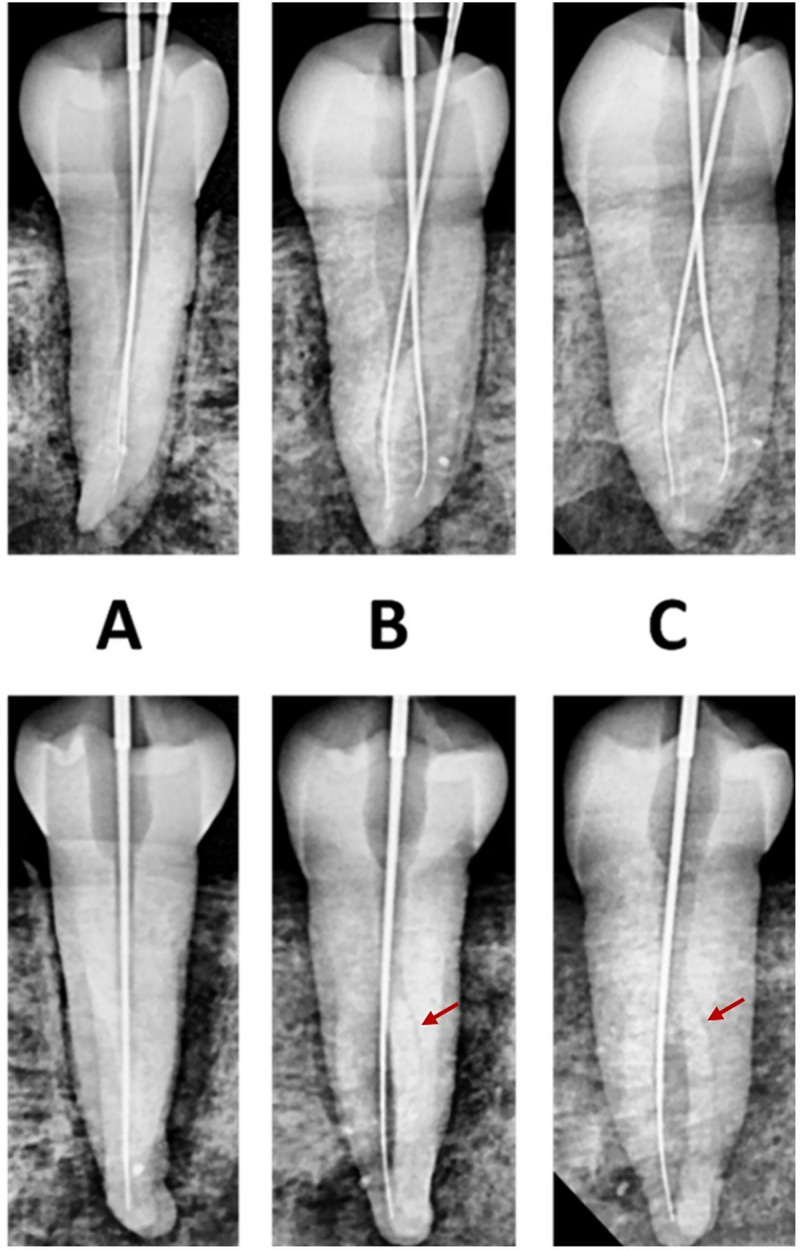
Radiographic images taken at different angulations in the pilot study showing detection (above) and missing of canals (below): A) at zero degrees, B) at 20 degrees, and C) at 40 degrees. Red arrow showing the missed canals.
